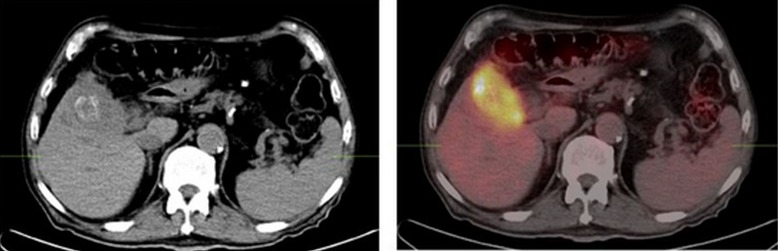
FDG-PET/CT These images demonstrate increased FDG uptake in the ​primary lesion infiltrating the liver.​
